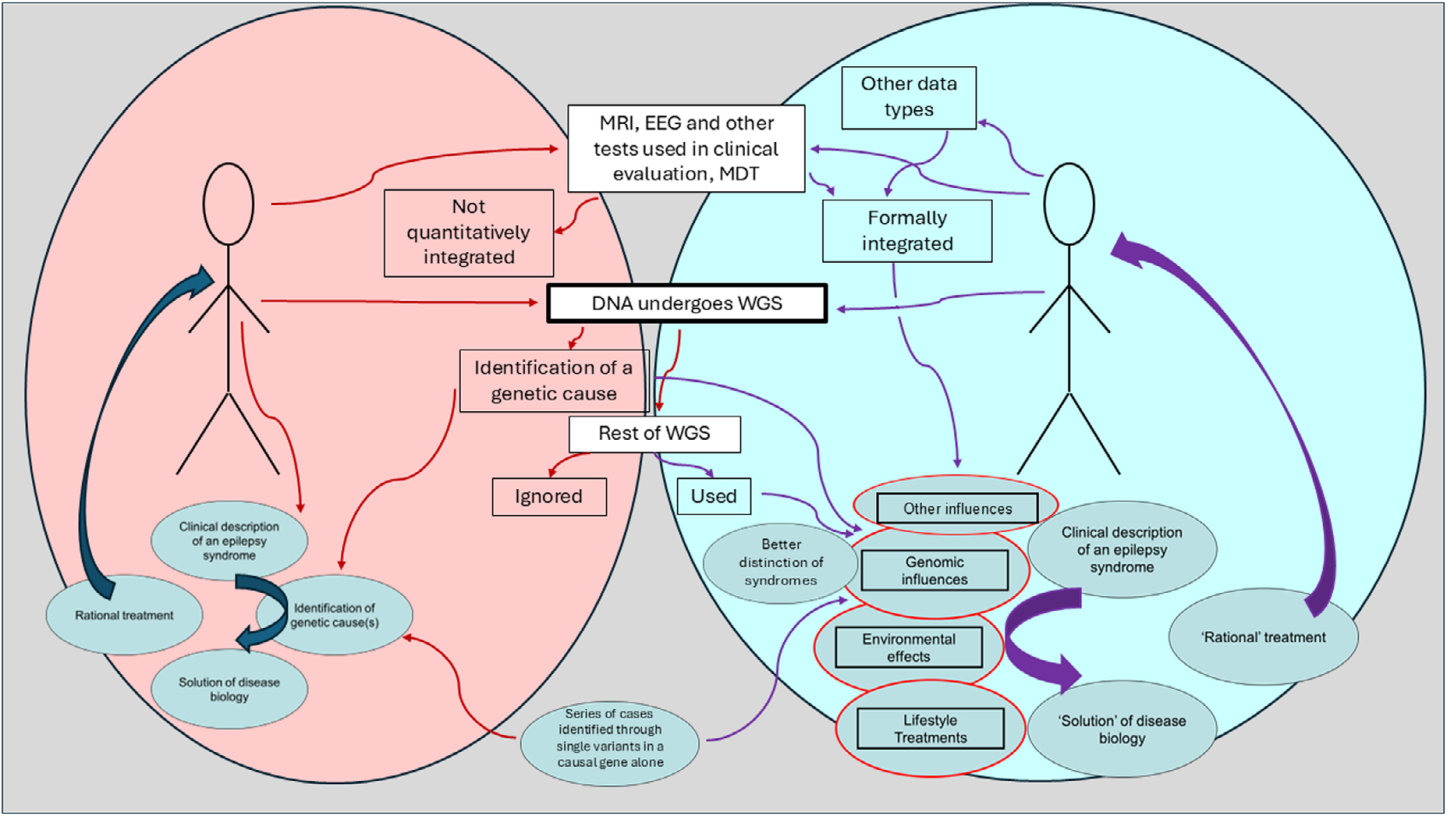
A current pattern of usage of genomic and other information is shown in the pink circle (left). Information from different modalities is typically not formally integrated but considered together in the expert review, for example, in a multidisciplinary team meeting. An alternative perspective considered in this review is shown in the blue circle (right). As much as possible of the remaining genome, not just the putatively causal variant, is used. It is formally integrated with other types of data to generate a better approximation of disease biology and rational treatment options; however, they are still shown within single quotation marks because even this approach is likely to fall short of a full understanding and treatment approach that tackles every difficulty faced by each individual. Abbreviations: EEG, electroencephalogram; MDT, multidisciplinary team; MRI, magnetic resonance imaging.
